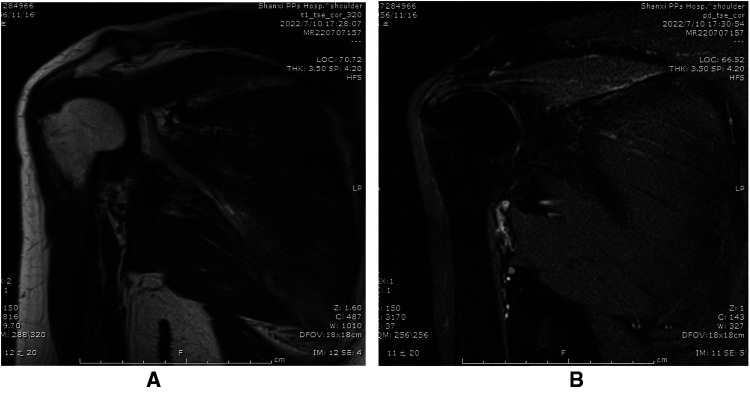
Preoperative MRI images: (A) calcified foci of supraspinatus tendonitis in preoperative T1WI. (B) Calcific tendonitis calcifications in preoperative T2WI.
